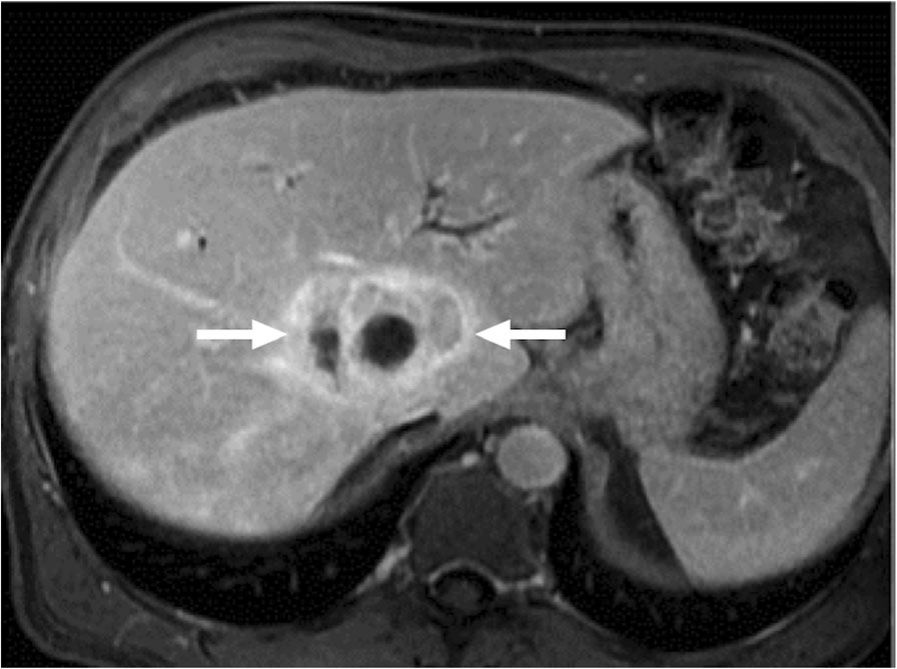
A 44-year-old male, previously healthy professional gardener on the island of Martha’s Vineyard, now presenting with cutaneous ulcer and lymphadenopathy on his arm, right upper quadrant pain, fever, and malaise. He was referred to MRI for a mass within the liver detected on abdominal US. Axial plane postcontrast image demonstrates an avidly enhancing, predominantly solid mass (arrows) adjacent to inferior vena cava and the caudate lobe. Acute titers for tularemia were elevated. Titers normalized and the lesion almost completely resolved on follow up MRI after doxycycline treatment
